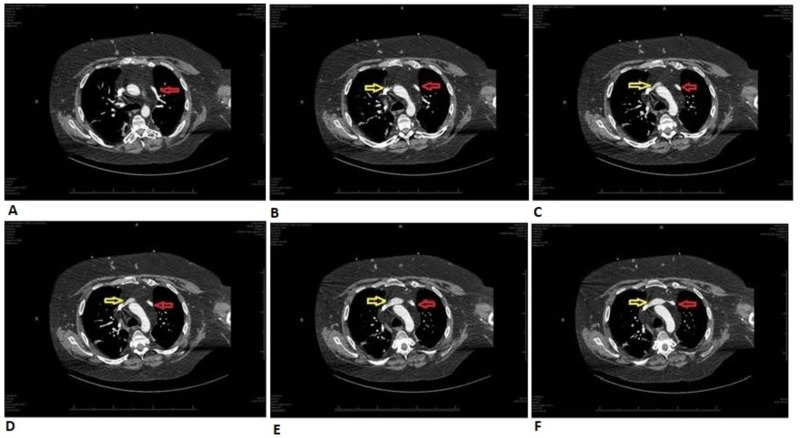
CT-angiogram axial plan showing PAPVC A CT-angiogram of the pulmonary arteries, axial plan, revealing the course (A-F) of the anomalous left superior pulmonary vein (red arrows) until joining the left brachiocephalic vein (yellow arrows).
